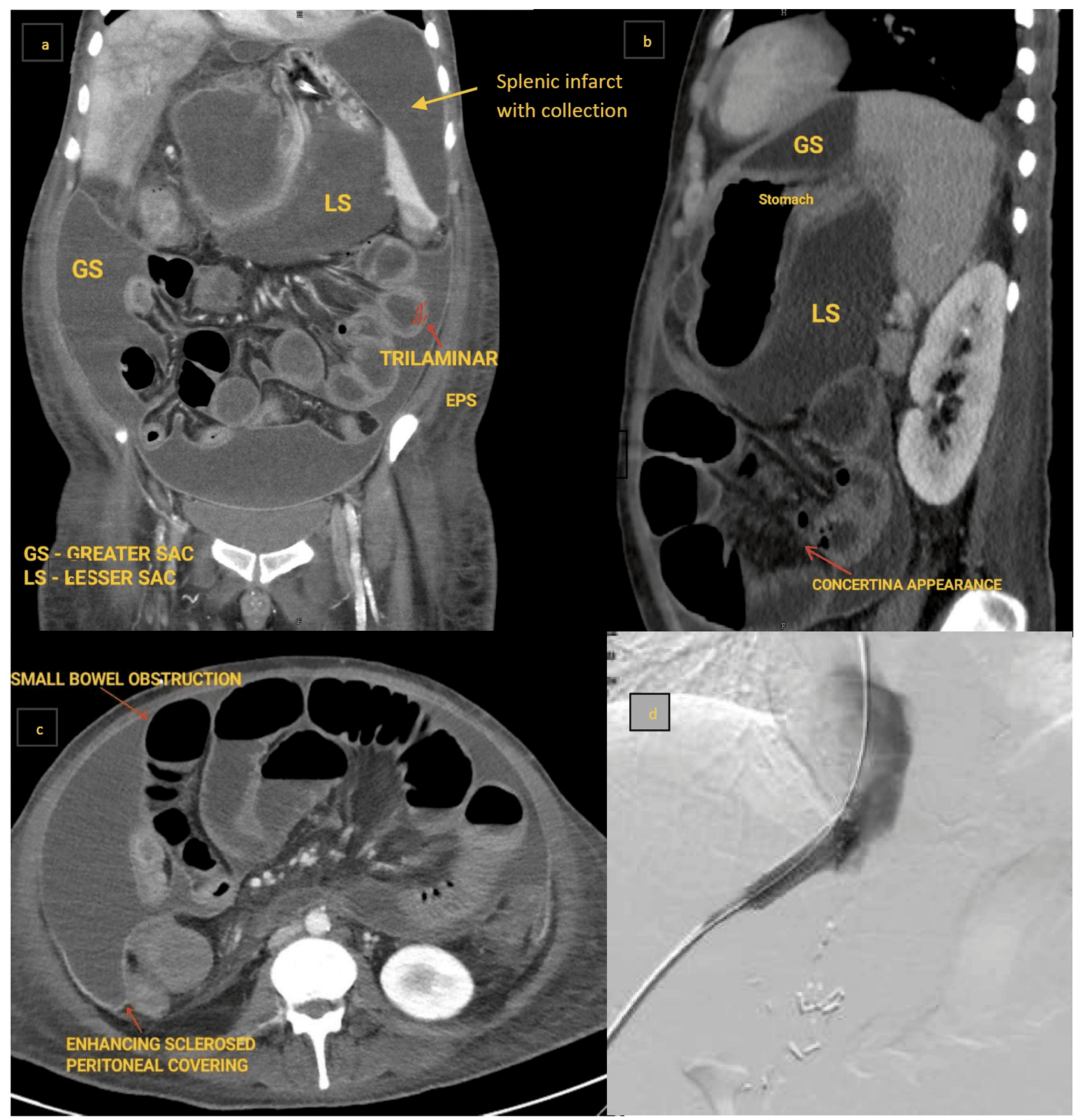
a: Contrast-enhanced CT (coronal) showing encapsulated fluid collections in the greater and lesser sacs, with perisplenic collections due to prior infarction. b: CECT (sagittal) showing encapsulated fluid collection. c: Axial CECT depicting encapsulation around small bowel loops clumped and conglomerated toward the root of the mesentery, with dilatation suggestive of subacute intestinal obstruction. d: Fluoroscopic image post-portal vein stenting. CT: computed tomography, CECT: contrast-enhanced computed tomography, GS: greater sac, LS: lesser sac, EPS: encapsulating peritoneal sclerosis
